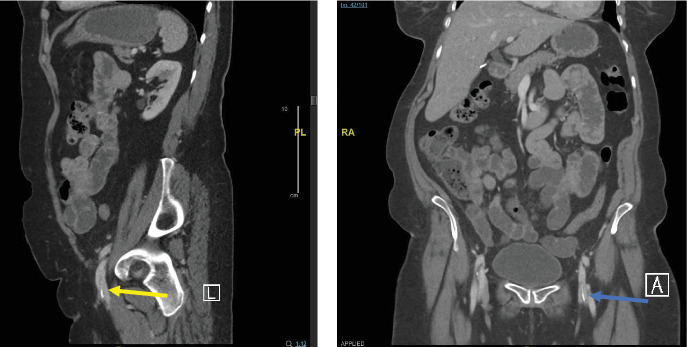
(A) The sagittal computed tomography scan of the abdomen showing a significant-sized linear hyperdense structure in the left common femoral vein (yellow arrow). (B) The coronal computed tomography scan of the abdomen reveals a clear linear hyper-dense structure in the left common femoral vein (blue arrow).
